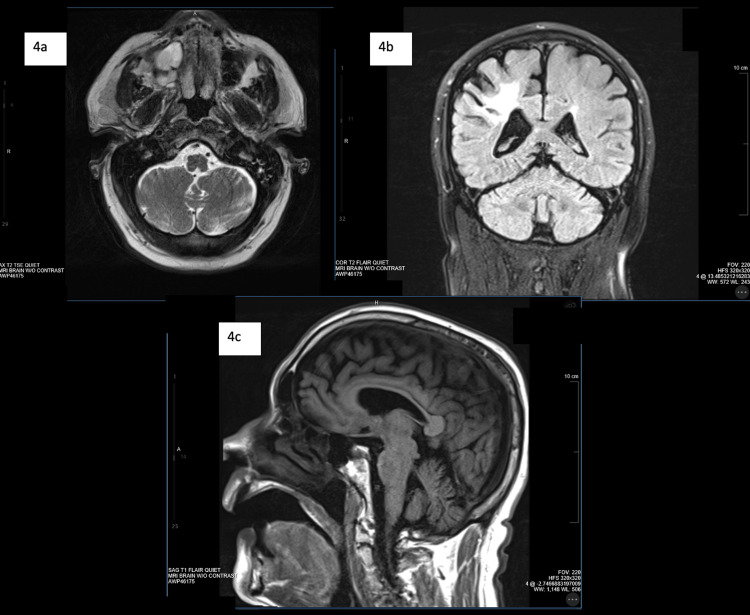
MRI Non-contrast Findings
